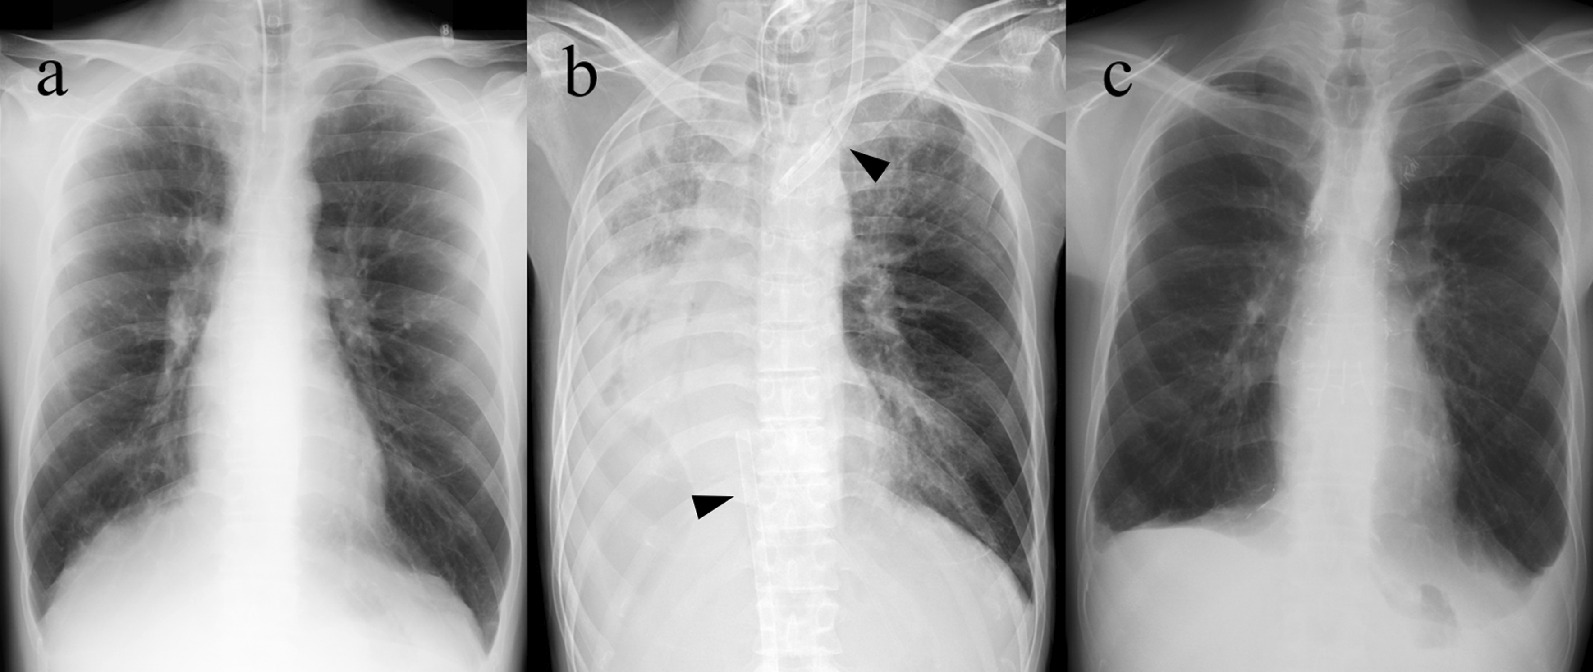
a Chest radiographs of the 27-year-old man with bronchiolitis obliterans caused by graft-versus-host disease after bone marrow transplantation. b Abnormal chest radiograph with consolidation due to pneumorrhagia in the right lung after long-term veno-venous extracorporeal membrane oxygenation. Two cannulas in the veins (arrowheads) and infiltration of the right lung are seen. c Chest radiograph at 18 months after bilateral lung transplantation
